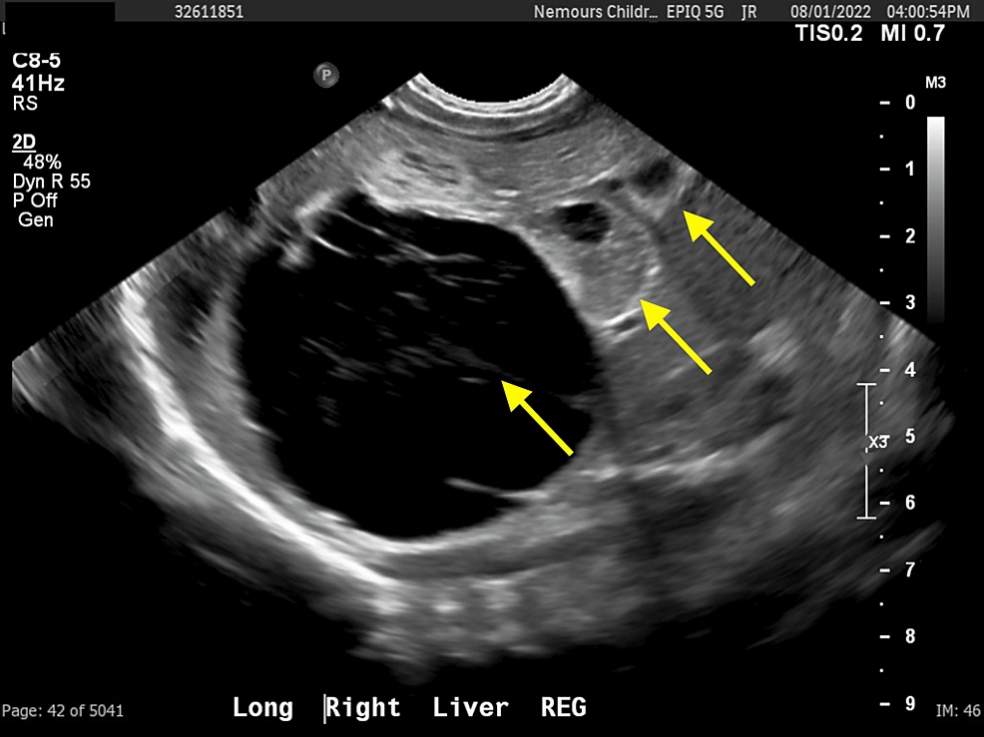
Sonographic image of the patient’s liver demonstrates a well-circumscribed, multiseptated, anechoic cyst surrounded by smaller mixed solid and cystic lesions
